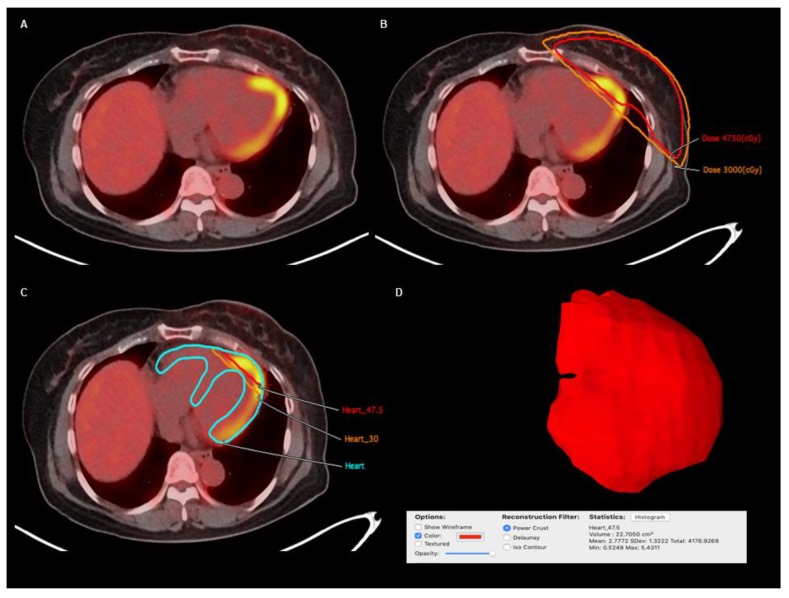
(A) Transaxial image and (B) transaxial image with dose-distribution on post-radiotherapy F-18 Fluorodeoxyglucose (FDG) positron emission tomography/computed tomography (PET/CT) in a 51-year-old woman with left breast cancer. (C) The structures of the whole myocardium (Heart) and myocardium irradiated with 30 Gy (Heart_30) and with 47.5 Gy (Heart_47.5) constructed from CT-stimulation images for radiotherapy were fused with the FDG PET/CT images. For each myocardial structure, the three-dimensional volume structure was automatically constructed and the mean FDG uptake of the volume structure was measured. (D) An example of three-dimensional volume structure of myocardium irradiated with 47.5 Gy in this patient is shown, displaying a mean standardized uptake value of 2.7772 for myocardium irradiated with 47.5 Gy.
